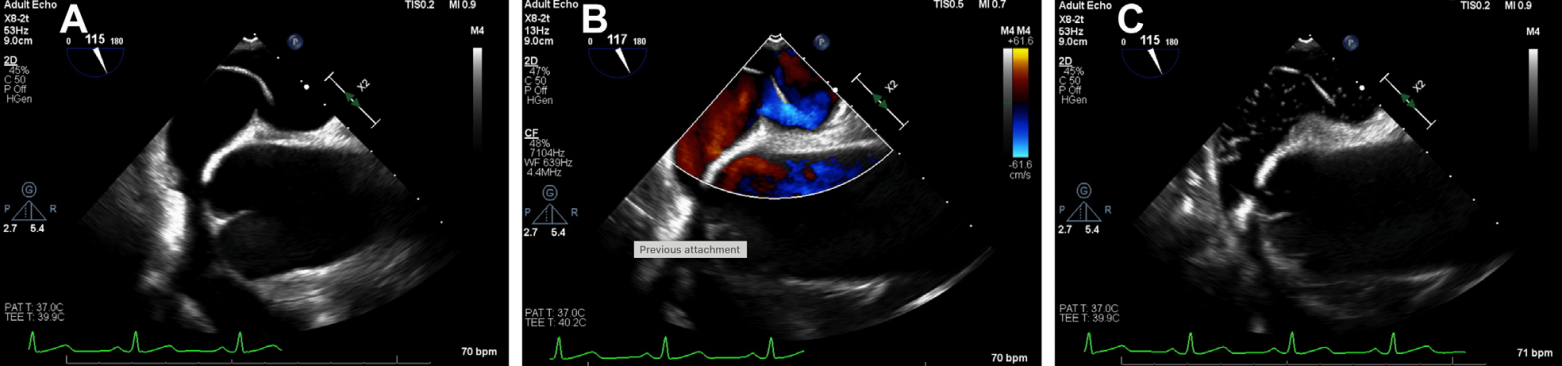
Transesophageal Echocardiography (A) Initial transesophageal echocardiography (TEE). (B) TEE with color Doppler. (C) TEE with bubble study. TEE reveals a mobile intra-atrial septum with right-to-left shunting.
